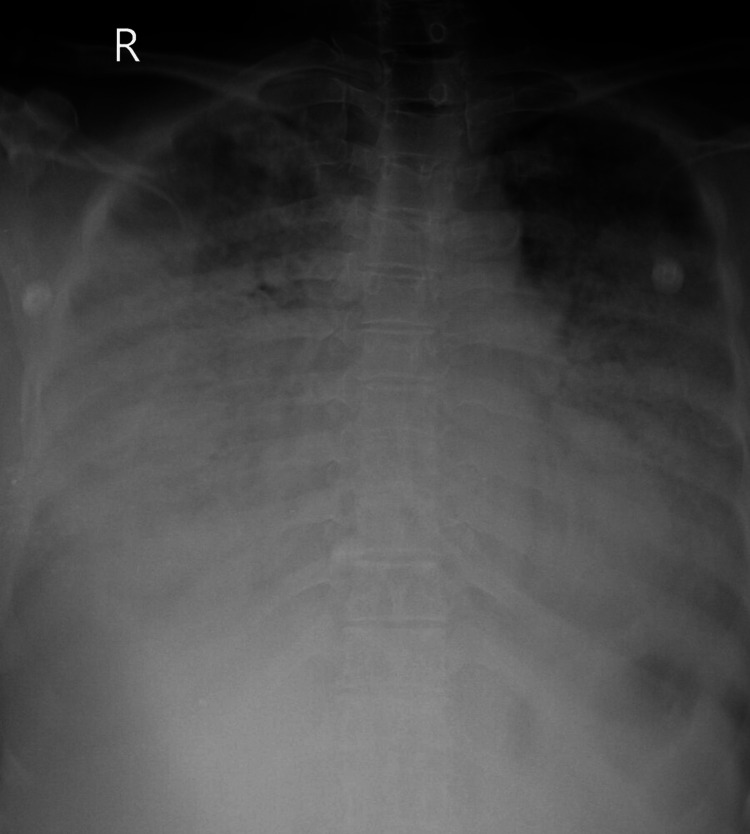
Chest X-ray on admission suggestive of bilateral extensive haziness (white-out lung).
